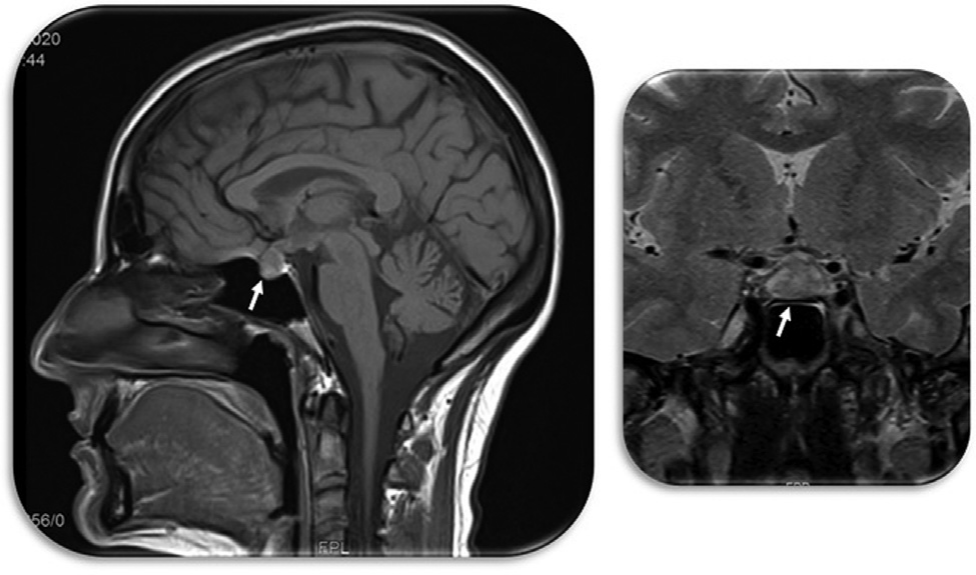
MRI showing pituitary apoplexy and arrows indicating the pituitary gland.
